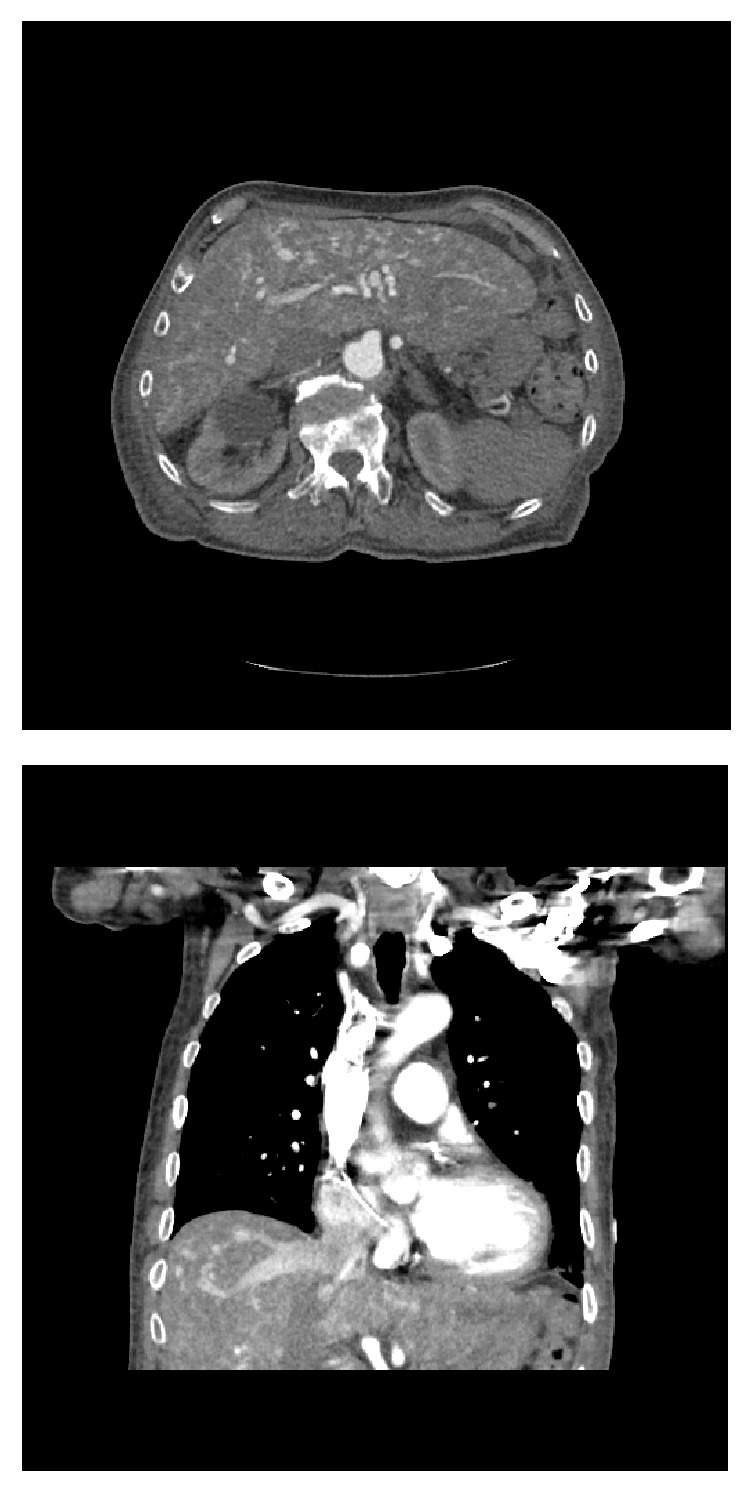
Computed Tomography in the axial (a) and coronal (b) arterial phase images showing an increased number of opacified hepatic arteries with early opacification of draining veins, consistent with multiple hepatic AVMs.
